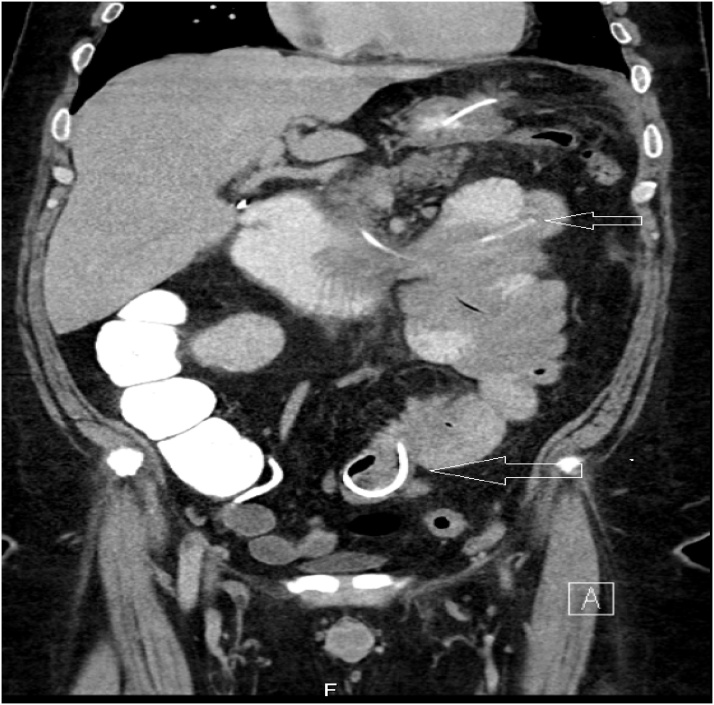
On Coronal abdomino-pelvic CT scan, the gastric band is localized in the upper jejunum (long arrow), with the catheter (short arrow) passing through the upper gastrointestinal tract. Thickened small bowel wall, as well as surrounding fat haziness is also seen.
